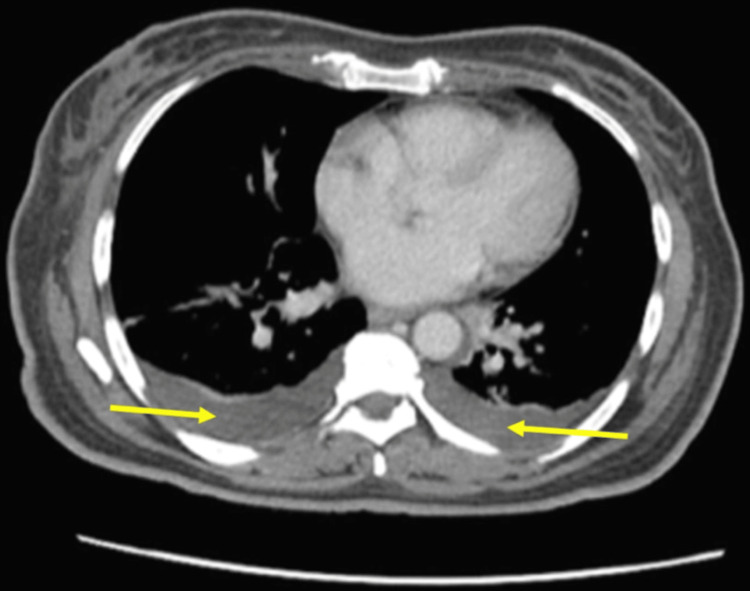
Contrast-enhanced CT of the abdomen and pelvis demonstrating third spacing of fluid with soft tissue anasarca (serious complication of AOSD) and small bilateral pleural effusions (denoted by the yellow arrows). AOSD: adult-onset Still's disease
